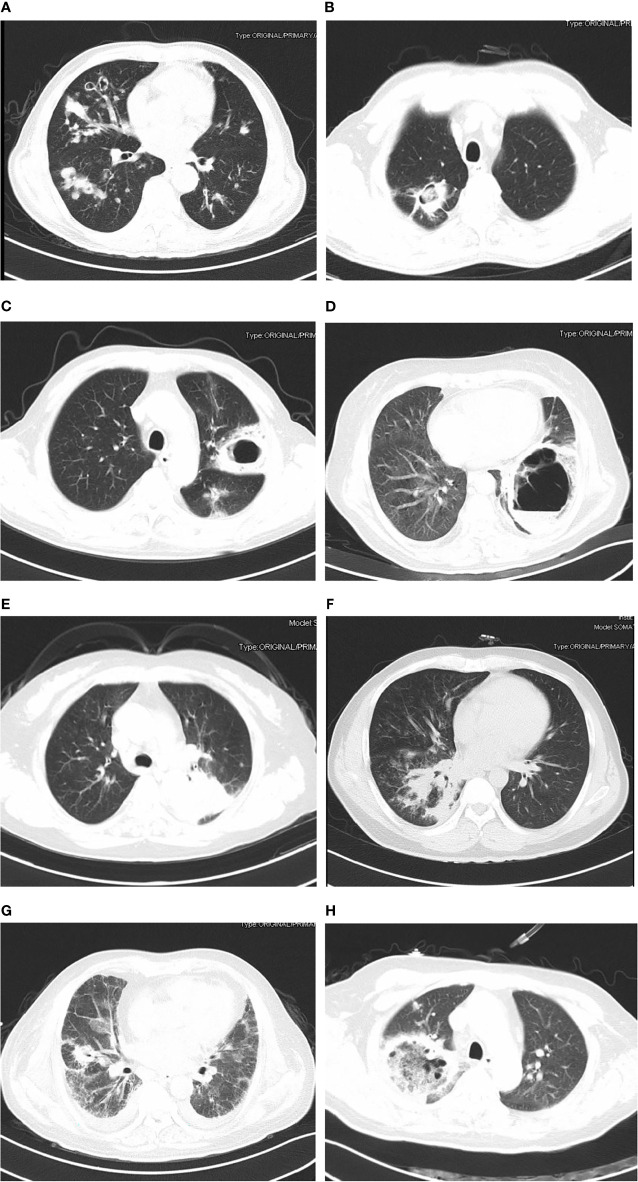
The findings of chest computed tomography of pulmonary mucormycosis (patients with good outcome: A, B; patients with worse outcome: C–H). (A) Multiple pleomorphic lesions in both lungs; (B) cavity with muralnodule (*Mucor* co-infecting with *Aspergillus*); (C) cavity in left lung; (D) thick-walled cavity shadow with gas-fluid level (*Mucor* co-infecting with *Klebsiella pneumoniae*); (E) mass shadow in the upper lobe of the left lung; (F) patchy shadow, nodules and consolidation in right lung; (G) cavity and ground-glass opacity; (H) reversed halo sign.
